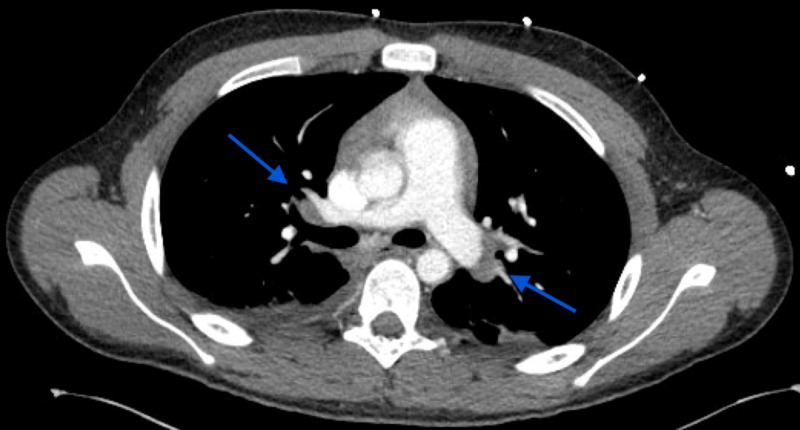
CT Chest Arrows indicate bilateral hilar lymphadenopathy
